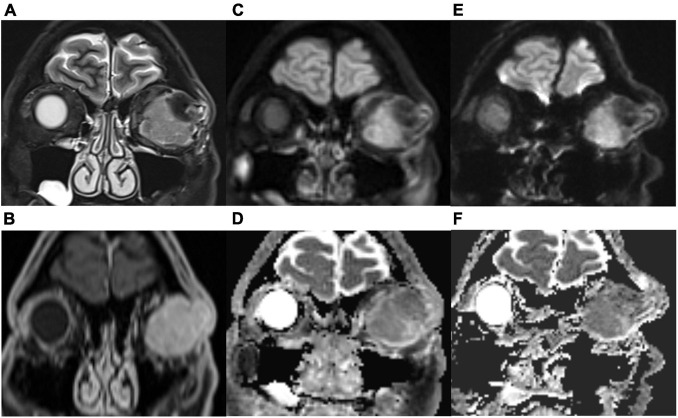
A 50-year-old male with left solitary fibrous tumor. Compared with the T2-weighted imaging (T2WI, A) images and contrast-enhanced T1WI (B), this lesion could be visualized clearly with TGSE-PROPELLER-DWI b1000 (C) and ADC map (D); no geometric distortions were seen. Slight distortions were seen with rs-EPI b1000 (E) and ADC map (F). More geometric distortions were observed in the frontal lobe and ethmoid sinus with rs-EPI.
